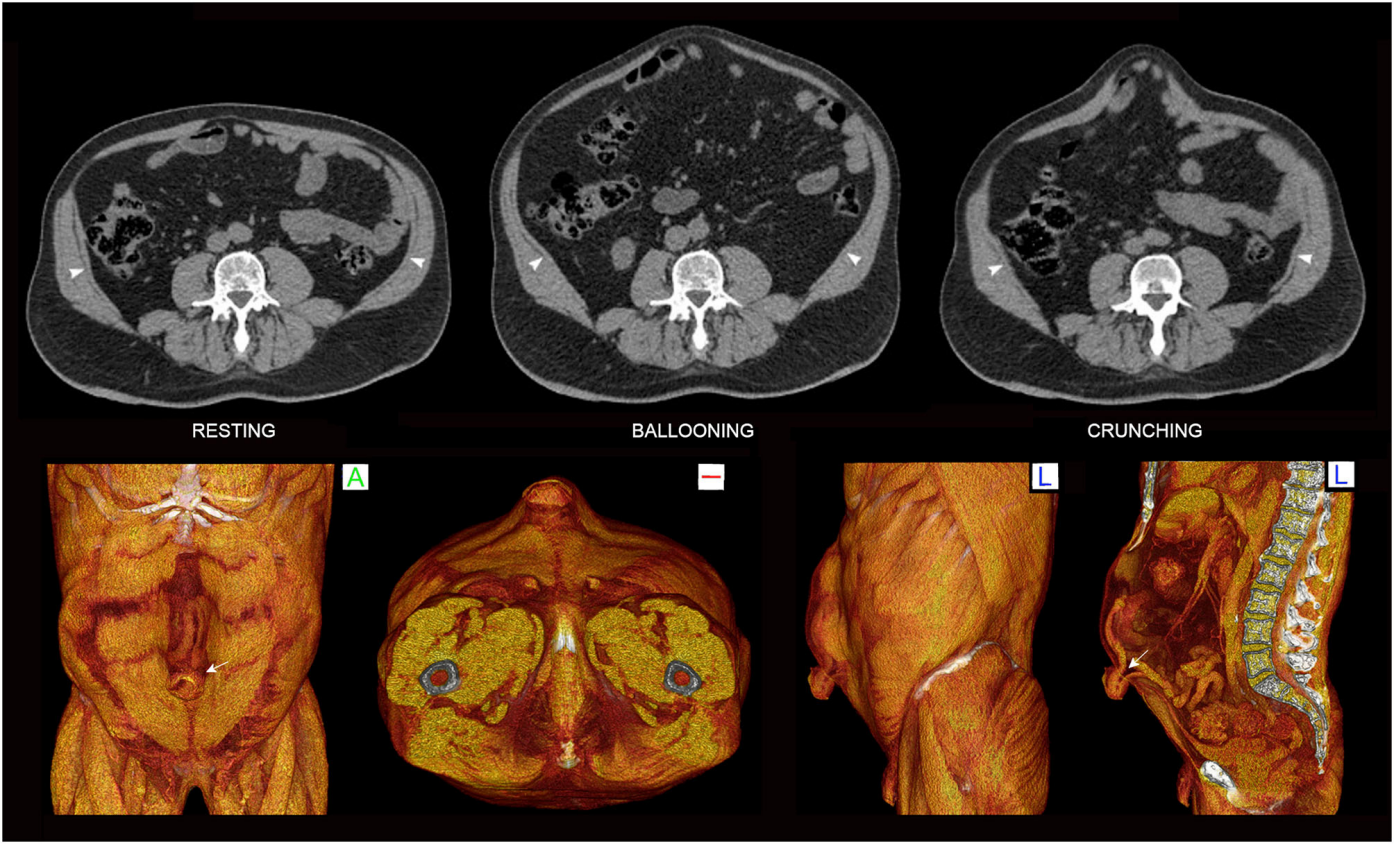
Diastasis rectus abdominis and superimposed umbilical hernia. Functional 3-phase 2D CT images obtained at supra-umbilical level (top) and crunching 3DVR images (bottom) show a small umbilical hernia with contained bowel (arrow) on a much larger background of diastasis rectus abdominis with associated prominent dysfunctional midline sagittal eventration bulge. Much of the bulge reflects poor TA function, appreciated as a lack of contractility on the ballooning and crunching phases (arrowheads) with associated eversion of the RA muscles along their medial edges. Note abrupt “angular shouldering” at the margins of the hernia (volume rendered images) vs. the continuous smooth contours at the margins of the dysfunctional bulge (2D images).
